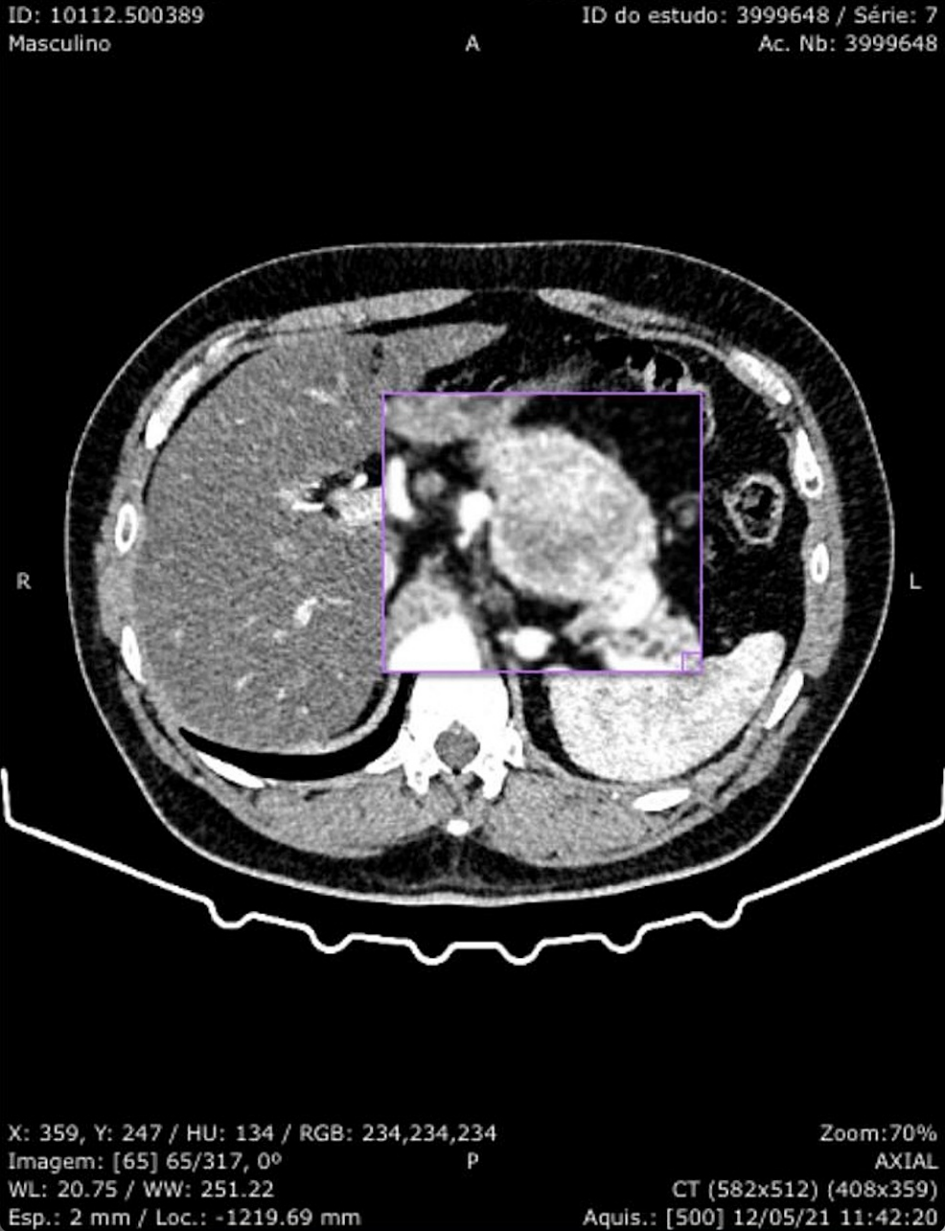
CT scan abdomen and pelvis with contrast; lesion enhanced. Lesion noted to the body of the pancreas.
